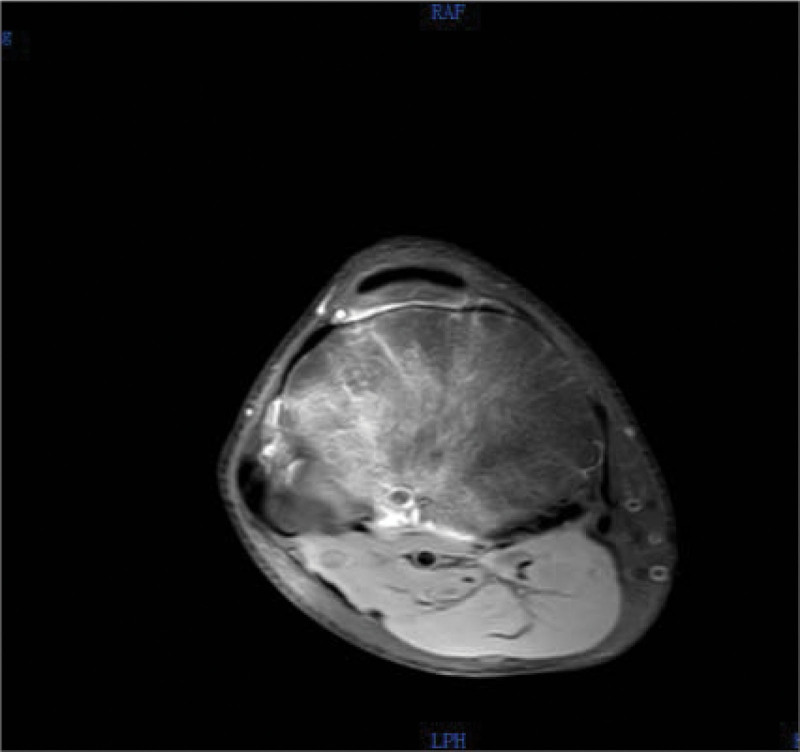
Preoperative MRI in transverse plane. MRI = magnetic resonance imaging.
